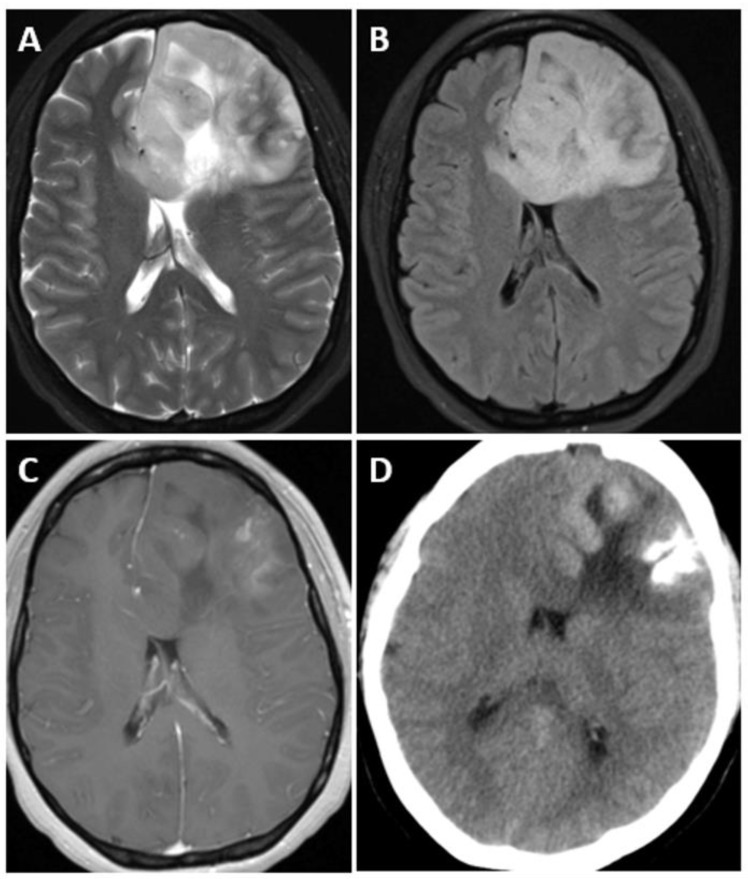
Axial T2-weighted (A), axial T2-weighted fluid-attenuated inversion recovery (FLAIR) (B), axial T1-weighted post-contrast(C), and unenhanced CT (D) through the level of the lateral ventricles in a 39 year old woman with oligodendroglioma, isocitrate dehydrogenase (IDH)-mutant and 1p/19q codeletion. Images demonstrate a heterogeneous left frontal lobe mass extending towards the right frontal lobe via the genu and proximal body of the corpus callosum. The mass demonstrates heterogeneous contrast enhancement and coarse calcifications.
